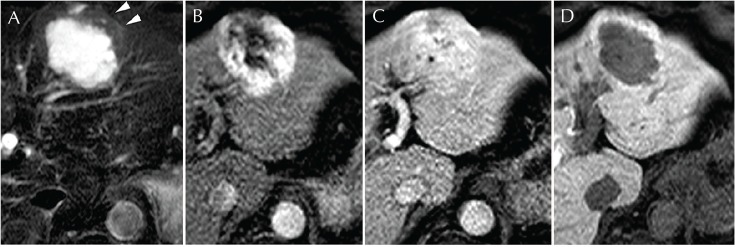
(A) Fat-saturated T_2_-weighted magnetic resonance (MR) image shows a nodule with strong hyperintensity and surrounding faint hyperintense area (arrowheads). (B, C) The arterial and portal phases of a gadoxetic acid disodium-enhanced MR images reveal a nodule with progressive, peripheral enhancement, and it appears hypointense with circumferential peritumoral hyperintense on (D) the hepatocyte phase.
